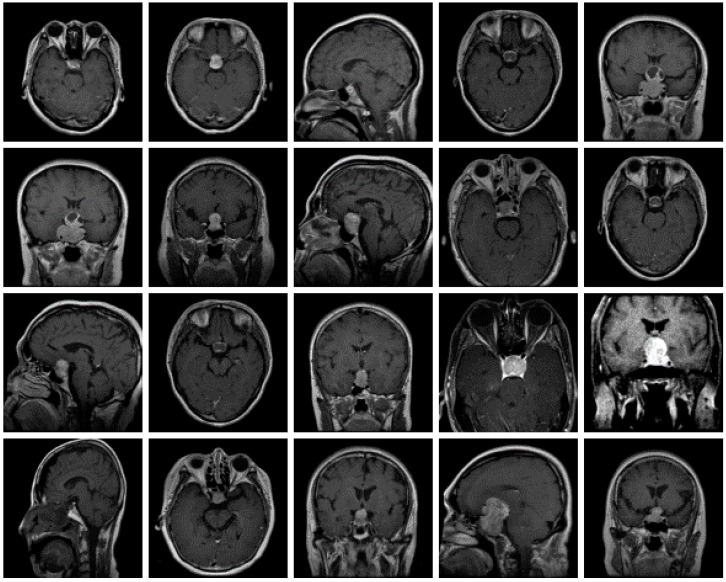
Examples of MRI scans of pituitary tumors.
